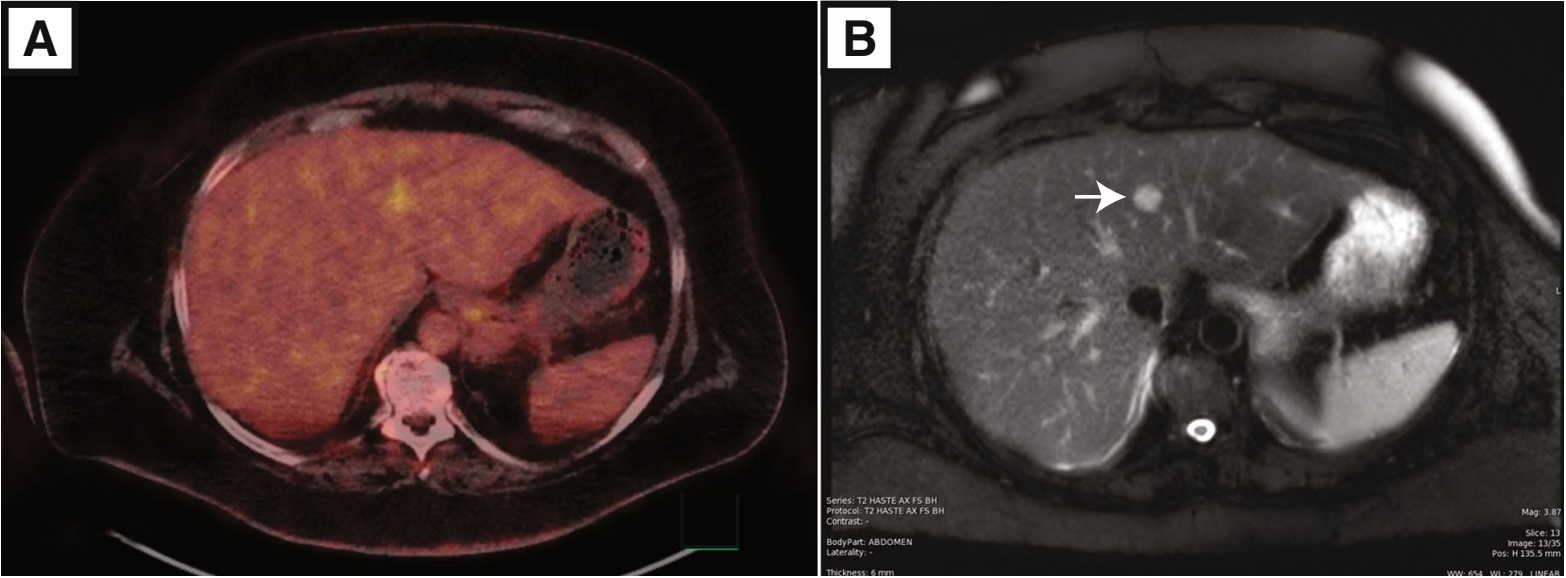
Imaging of the liver lesion. PET-CT (a) showed heterogenous liver uptake with a focus in the medial left lobe. T2-weighted MRI (b) confirmed a discrete 1.8 cm lesion suspicious for metastasis (*arrow*). This was later confirmed by biopsy
